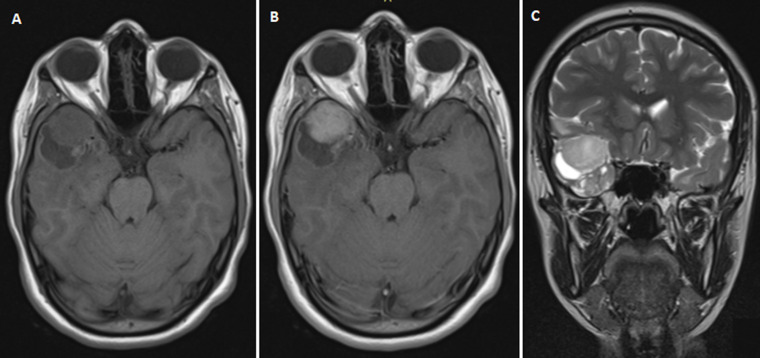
preoperative magnetic resonance imaging of the brain showing an extra-axial lesion in the right temporal fossa with two components solid and cystic (A) isointense on T1 weighted-images, (B) heterogeneously enhanced in T1 weighted-images, and (C) isointense on T2 weighted-images
